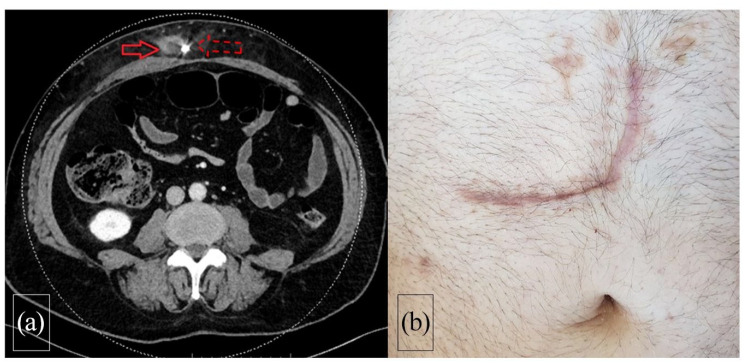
(a) Thoraco-abdominal CT shows important abscess (solid arrow) along the LVAD
